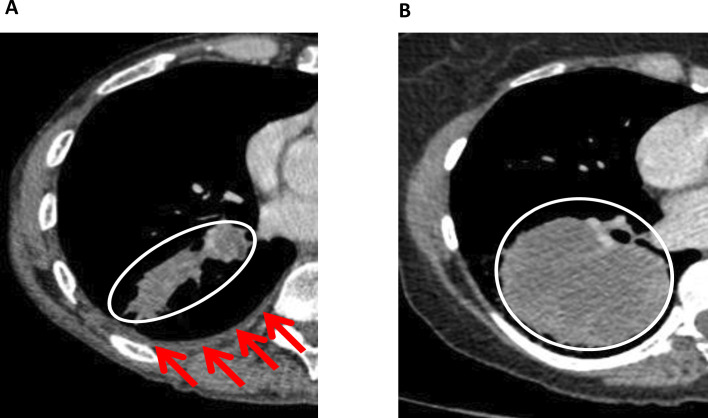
Minimal pleural effusion (mini-PE) examples. Both panels show axial plane CT images in patients with non-small cell lung cancer (NSCLC). (A) A T2b N1 M0 (stage 2A) NSCLC with associated Mini-PE (red arrows). Based on retrospective data, the HR for death in this case is 2.24 relative to T2b N1 without Mini-PE.^3^ (B) A T3 N1 M0 (stage 2B) NSCLC without Mini-PE. Both patients have potentially radically treatable disease (circled).
